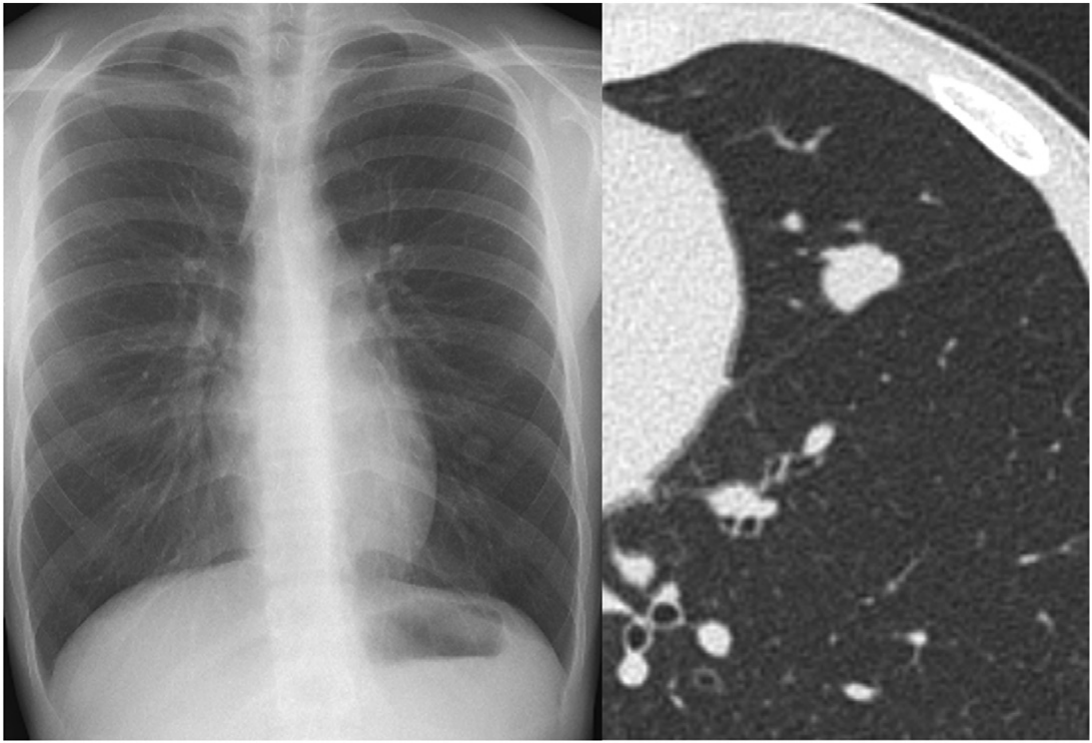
(Left) The chest roentgenogram showed an abnormal shadow in the left lower lung field. (Right) The computed tomographic image showed a 13-mm nodular shadow with suspected interlobar pleural indentation.
